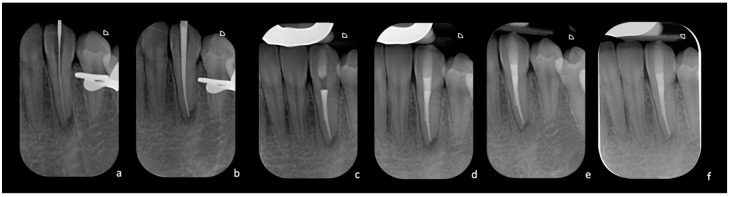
(a) Intra-operative X-ray of the K-file inserted at WL after locating the canal. (b) Intra-operative X-ray with the gutta-percha cone test. (c) Post-operative X-ray with completed root canal filling. (d) Radiograph taken after completing the coronal seal. (e) Radiographic control at three months with signs of improvement of the apical radiolucency. (f) Radiographic follow-up at two years with complete healing.
